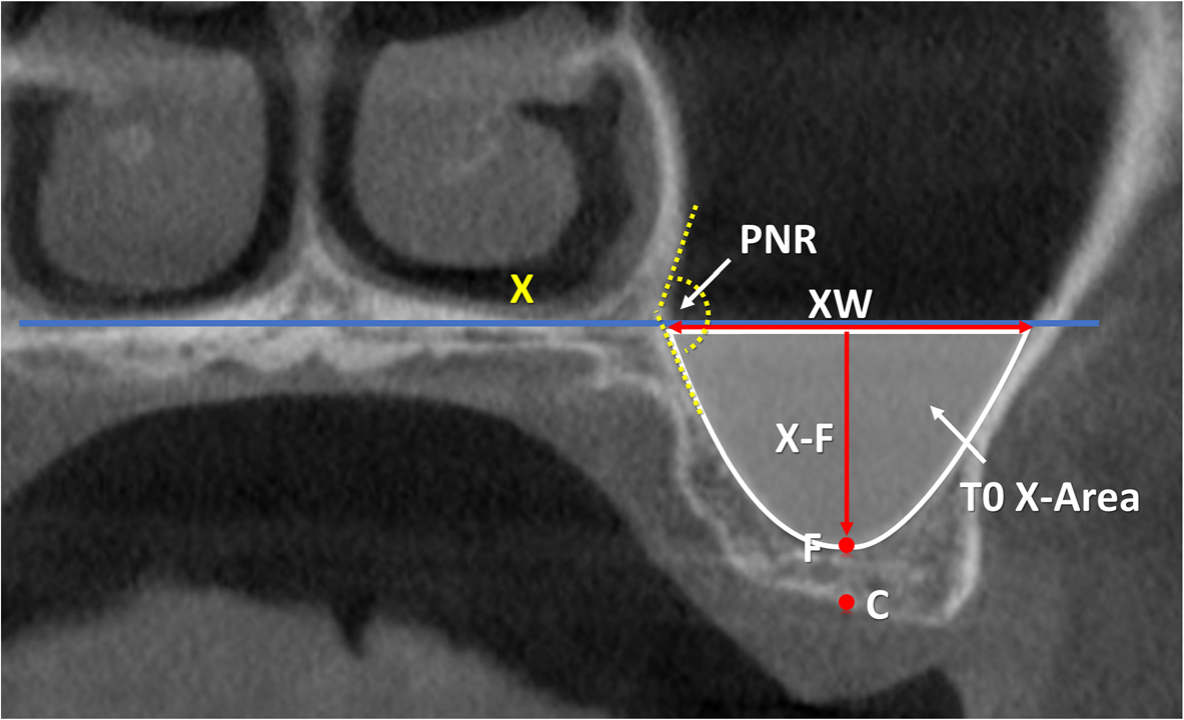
T0, coronal view of a no-membrane CBCT; X, line drawn following the floor of the nose; C, center of the bony crest; F, base of the sinus floor; PNR, palatal-nasal recess. X-F, nasal floor height; XW (sinus width), distance evaluated on the line X between the two intersection points with the medial and lateral sinus bone walls; T0 X-Area, area delimited by the sinus bone walls and the line X. The PNR angle is indicated in yellow
